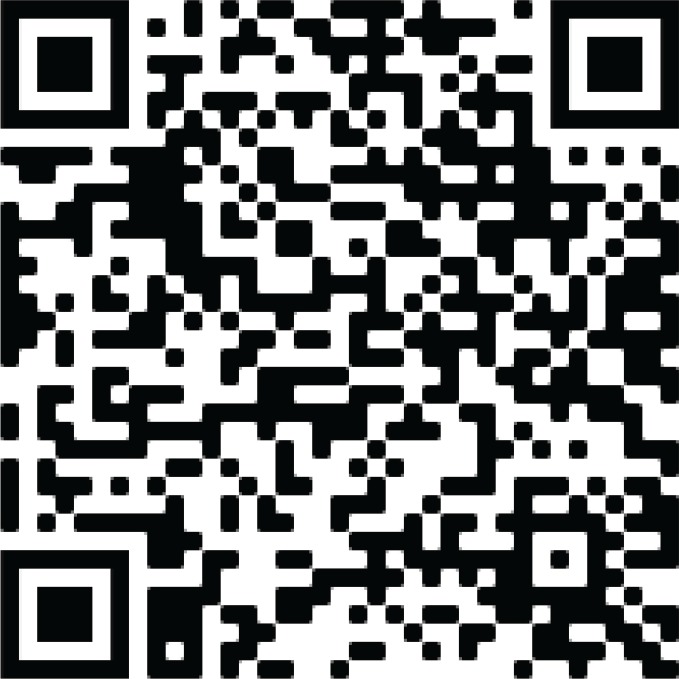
Epicardial echocardiography.
